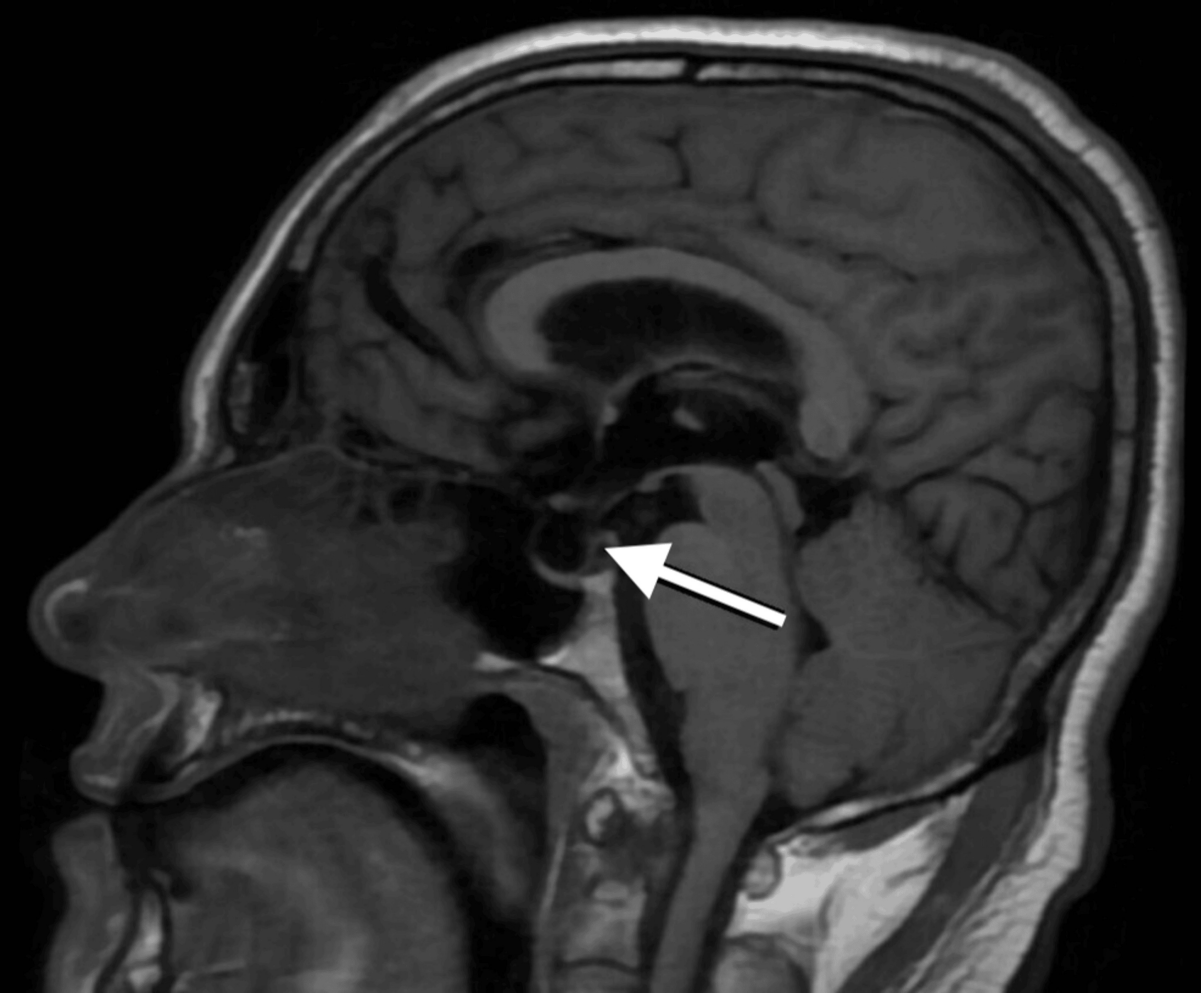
T1-weighted MRI demonstrating an empty sella (white arrow)
